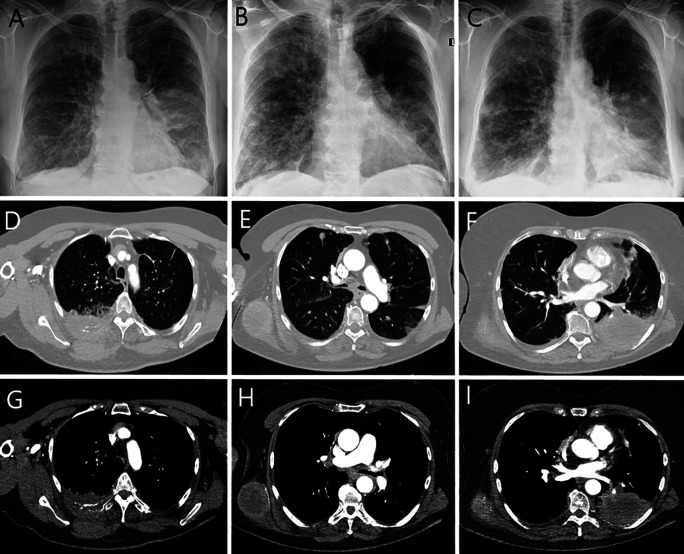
Chest radiograph (A-C): Diffused, patchy opacities and consolidations, bilaterally (more in right lung). Rounded mass in the left lung at the level of the lower pole of the hilum (A). Partial regression of opacities during (B) and after anti-inflammatory treatment (C). Chest computed tomography (D-F lung window, axial scans; G-I abdomen window, axial scans): mass of the posterior chest wall on the right side size 7.5×3.4 cm, infiltrating the posterior segment of the fifth rib (D, G); rounded metastasis up to 10 mm in diameter in both lungs, tumor in the right dorsal muscle size 6×5 cm (E, H); tumor in the 6, 9 and 10 segments of the left lung size 9×6×6 cm, infiltrating eighth and ninth rib, with bone destruction (F, I).
